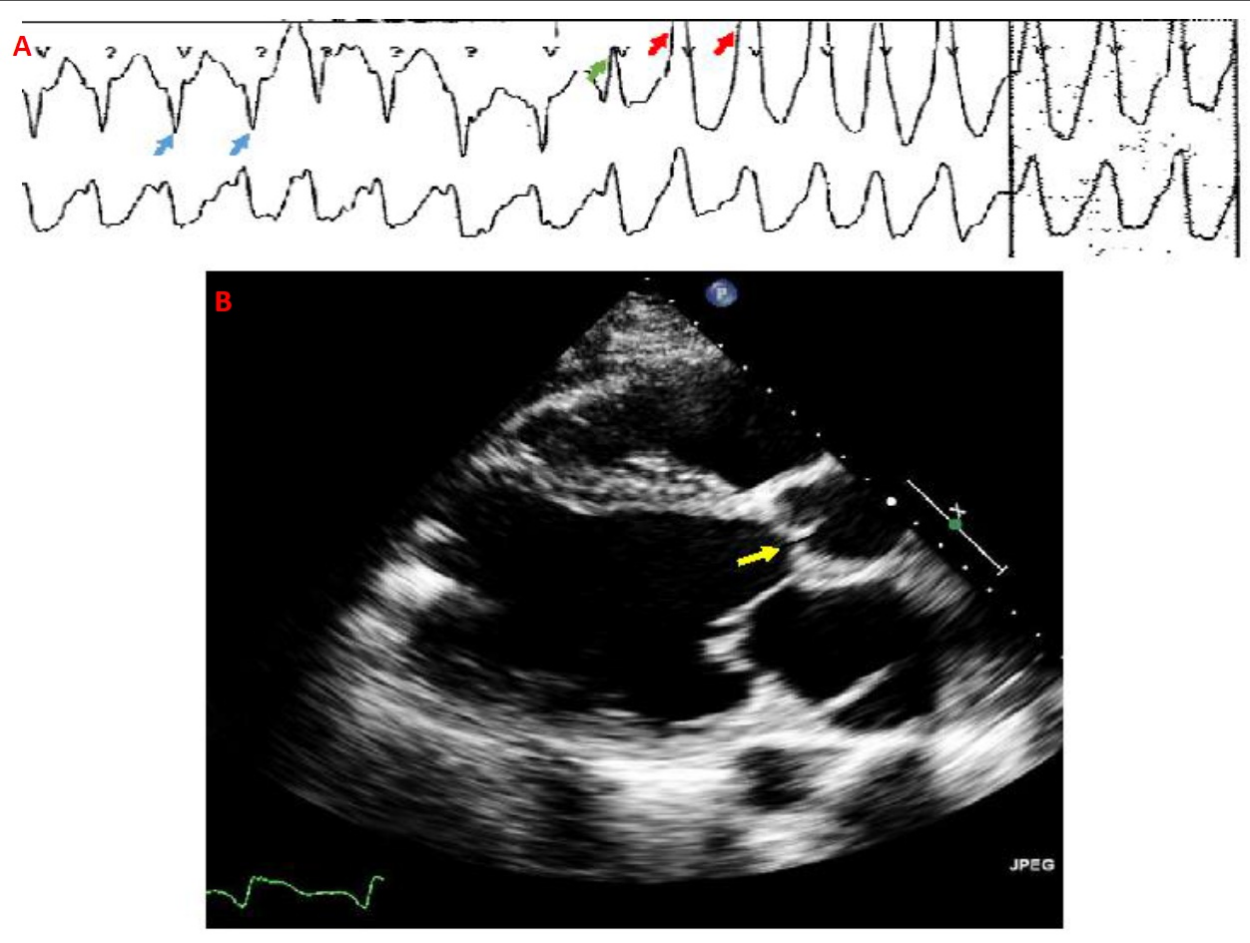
Telemetry (A) and Echocardiography (B) A) Telemetry strip showing ventricular tachycardia Blue arrows: paced rhythm, Green arrow: fusion beat, Red arrows: Ventricular tachycardia B) Left parasternal long-axis view showing lack of opening of both aortic cusps (yellow arrow) in systole
